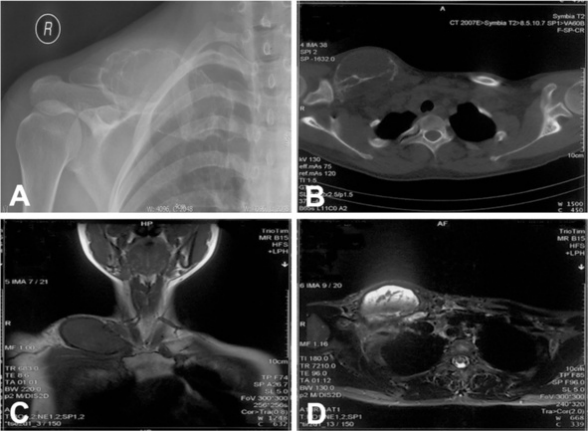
A 21-year-old woman with clavicle Ewing’s sarcoma. a X-ray film showed osteolytic lesion at the right clavicle. b CT showed large lytic lesion involving the whole clavicle. c The tumor showed low intensity on T1-weighted MRI. d The tumor showed hyper intensity on T2-weighted MRI
